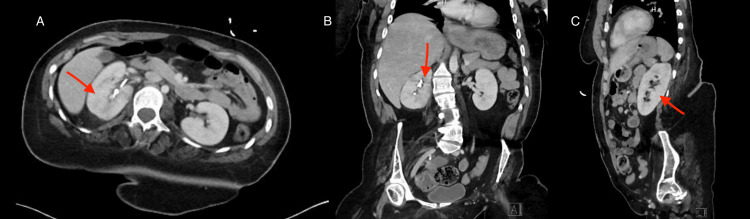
Follow-up contrast-enhanced CT demonstrating interval improvement of right-sided pyelonephritis. (A) Axial contrast-enhanced CT improving cortical enhancement with mild perirenal fat stranding. (B) Coronal reconstruction of multiple renal calculi in the right pelvis, resolving pyelonephritis. (C) Non-obstructive right nephrolithiasis and improving cortical inflammatory changes consistent with pyelonephritis.
